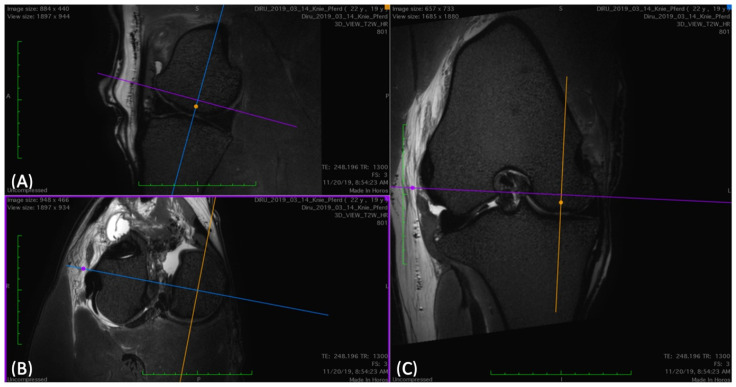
(A) Sagittal, (B) transverse, and (C) dorsal view of 3D T2-weighted view images of a stifle, where the plane for the regions of interest (ROIs) is determined for the medial femorotibial joint. To have a sagittal view directly through the middle of each condyle, the dorsal plane (blue) was aligned in parallel to the caudal horn of the meniscus and the transverse (purple) parallel to the joint space. In the dorsal (C) and transverse (B) views, the sagittal plane (yellow) was placed through the center of the condyle. This was repeated for lateral measurements.
